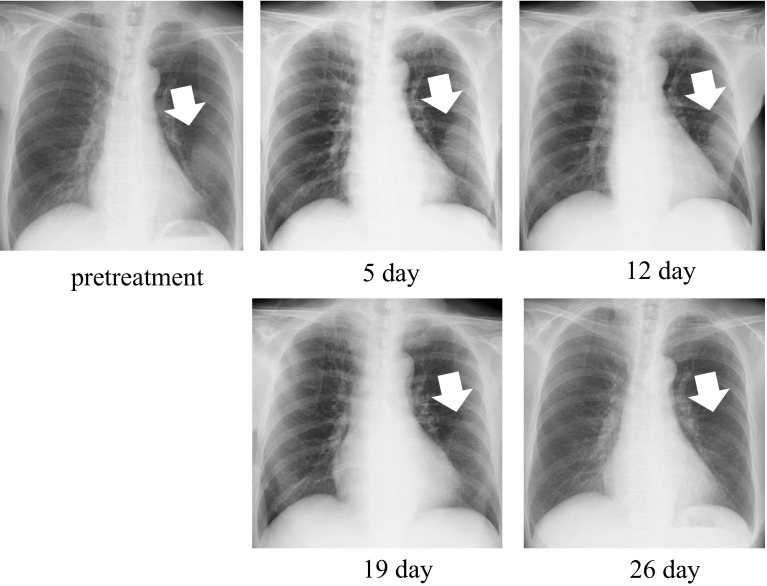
Response of a primary mucosal melanoma metastasis to nivolumab after the first treatment. The pretreatment chest radiographic image shows rib metastasis. Meanwhile, the chest radiographic image obtained 5–26 days after the first treatment shows that the costal tumor (*arrow*) had resolved
